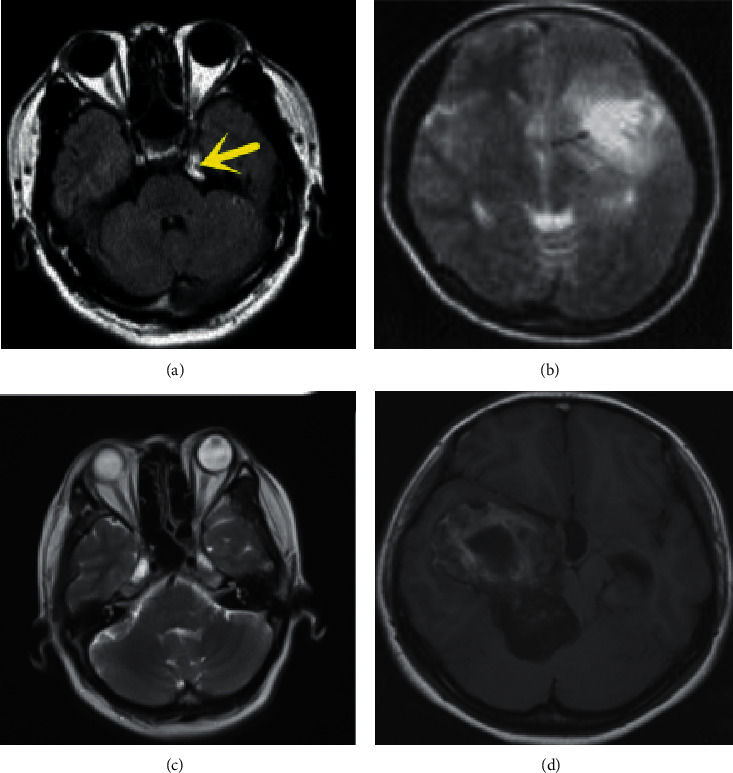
MRI images of (a) a non-HIE child and (b–d) children with HIE.
